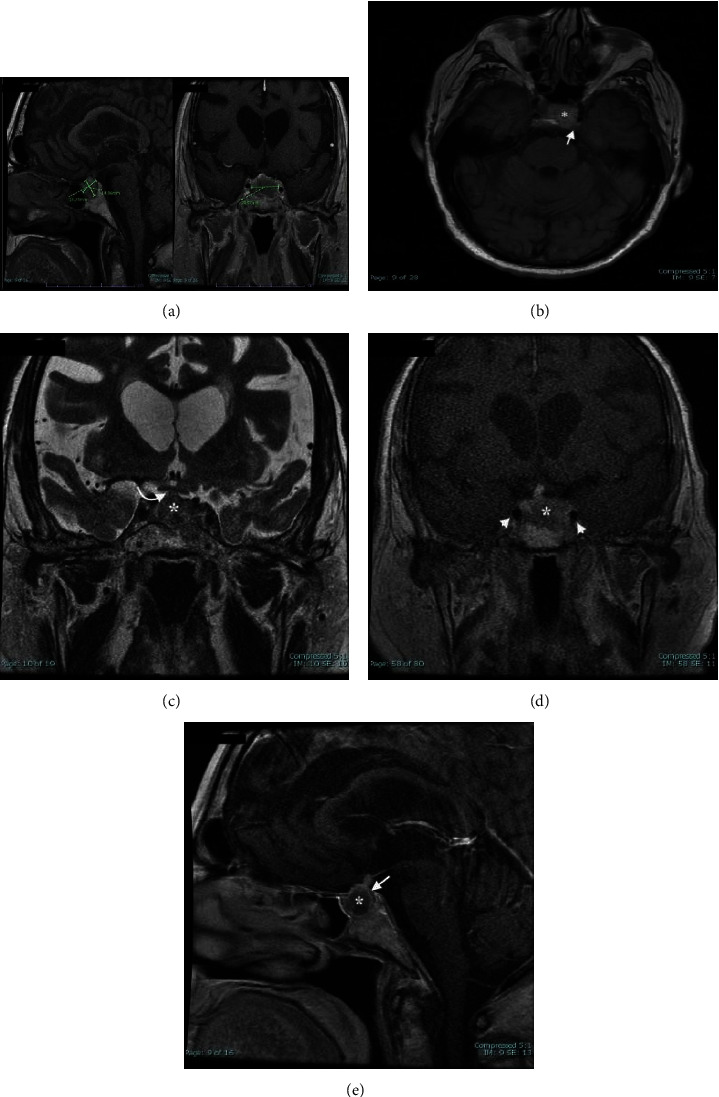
MRI brain images. (a) Sagittal and coronal T1 weighted sequence of the sellar region demonstrates a 2.5 cm × 1.5 cm × 1.5 cm heterogeneously T1 hyperintense mass expanding the sella turcica. Note the patchy T1 bright signal throughout the mass, consistent with subacute hemorrhage. (b) Axial T1 sequence of the brain again demonstrates the heterogeneously T1 hyperintense mass (asterisk) extending in to the left greater than right cavernous sinuses (arrow). (c) Coronal T2 sequence shows the T2 hypointense mass (asterisk) with minimal mass effect upon the optic chiasm (curved arrow). (d) Dynamic coronal contrast sequences show the hypoenhancing left sellar mass (asterisk). Note the preserved carotid flow voids within the cavernous sinus (arrowheads). (e) Delayed sagittal contrast sequence shows the hypoenhancing mass (asterisk) with claw-like hyperenhancing pituitary parenchyma along the periphery (arrow).
